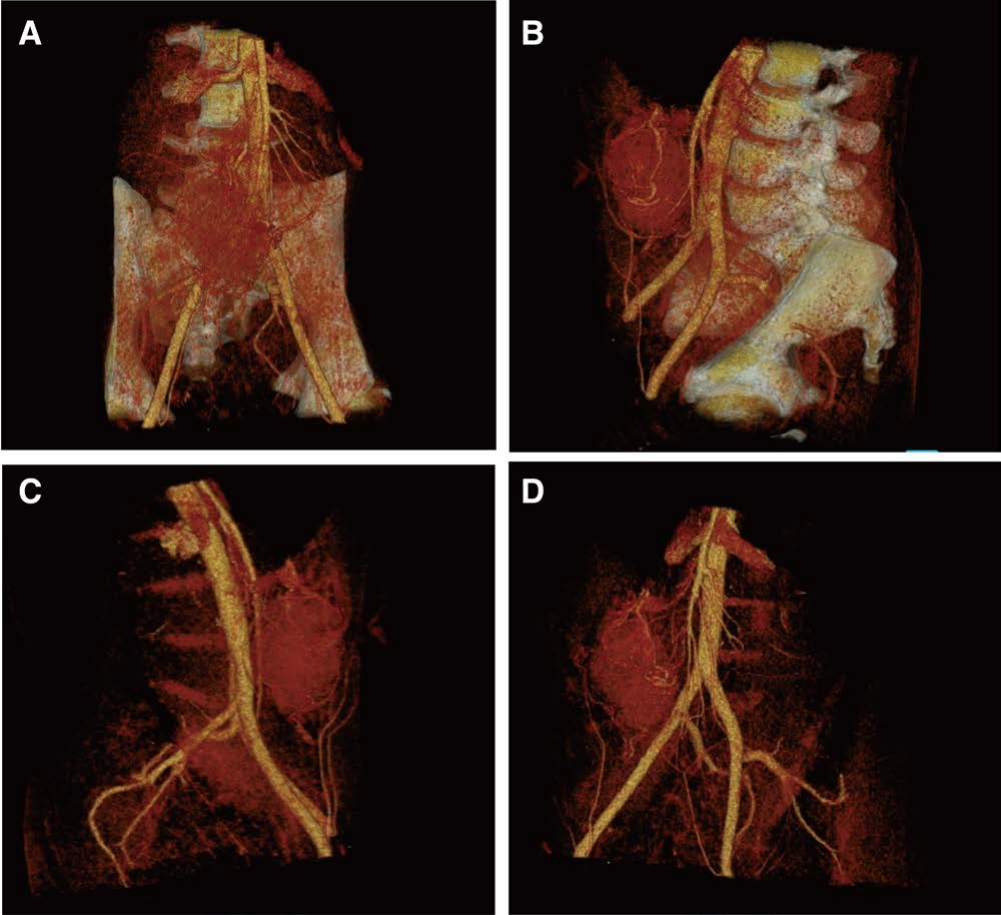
(A–D) Three-dimensional reconstruction images were performed from CT images to demonstrate tumor size and location in anterior and lateral views, respectively. CT = computed tomography.
